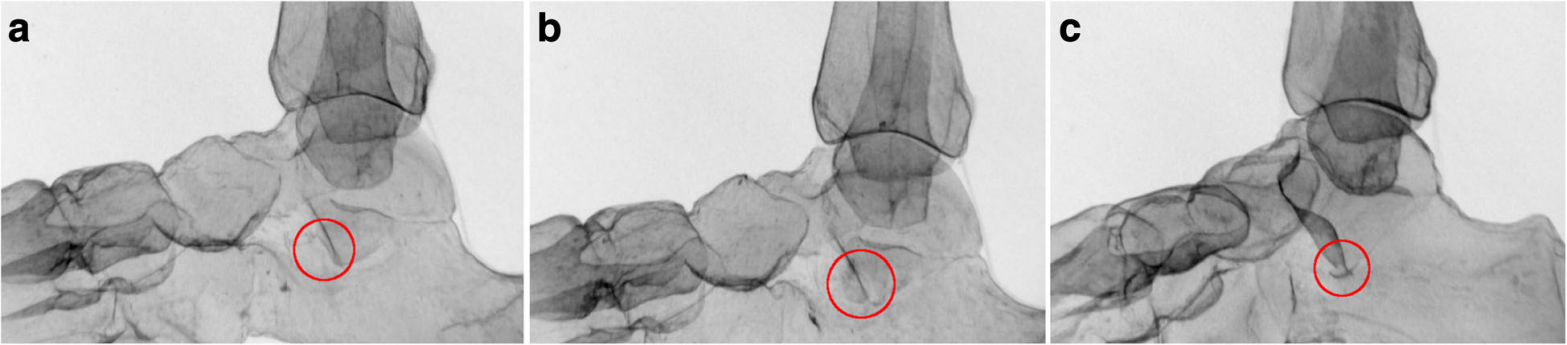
The V sign on lateral radiographs, as demonstrated in the sawbone models. The *circles* indicate the location of the V sign. a Positive V sign; type A fracture. b Positive V sign; type B fracture. c Positive V sign; type C fracture
